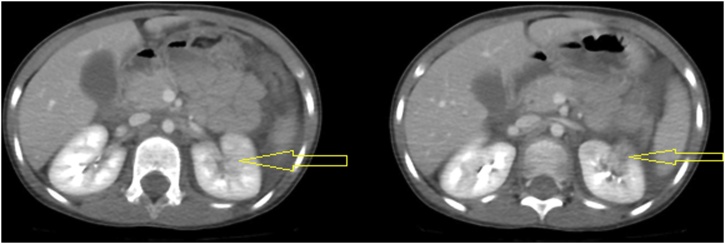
Case 2, CT trauma revealed left renal laceration and contusion (yellow arrows).
